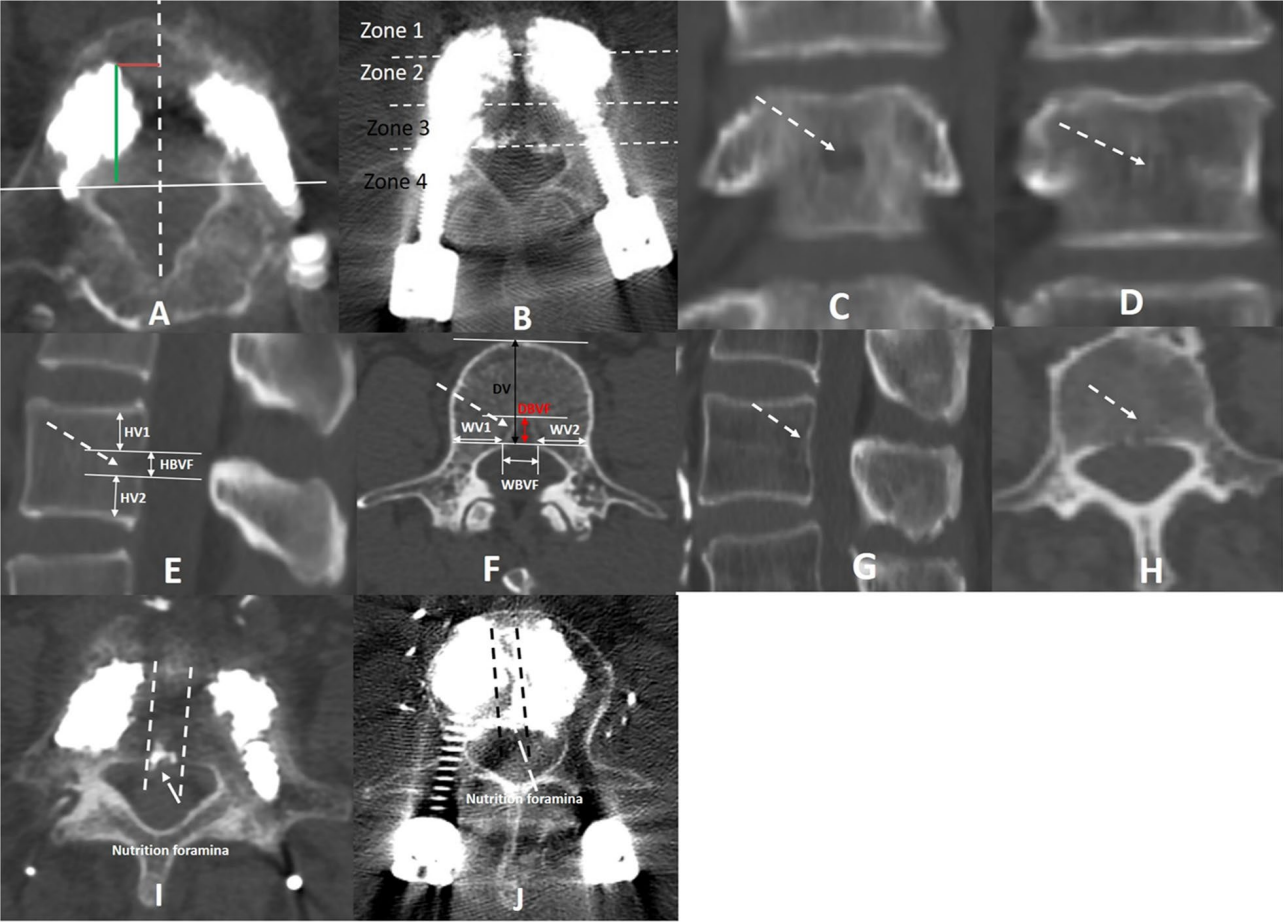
A On an axial CT scan, the axial location of pedicle screw implantation in the medio-lateral direction (indicated by the red line) and the anterior–posterior direction (indicated by the green line) within the vertebral body. B On CT axial view, the distribution of cement within the vertebral body was classified into four zones. C–F. A magistral form of basivertebral foramen (a large, single centrally located foramen with a width/height of ≥ 5 mm). D–H. A disperse form of basivertebral foramen (multiple small nutrient foramina). I. Local ECL on axial CT image (cement meniscus only at the nutrition foramen). J. Extensive ECL on axial CT image (cement mass surpassing the limits of the nutrition foramen in the posterior wall of the vertebral body, or leakage in the canal measuring over 2 mm in thickness). ECL epidural cement leakage
